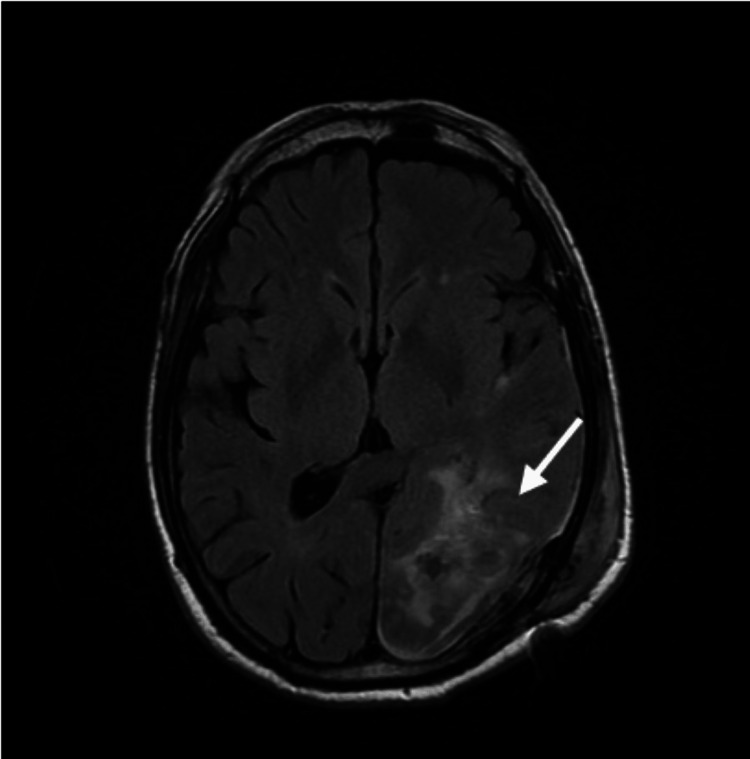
T2 sequence of axial cut of head MRI after surgery MRI: magnetic resonance imaging
